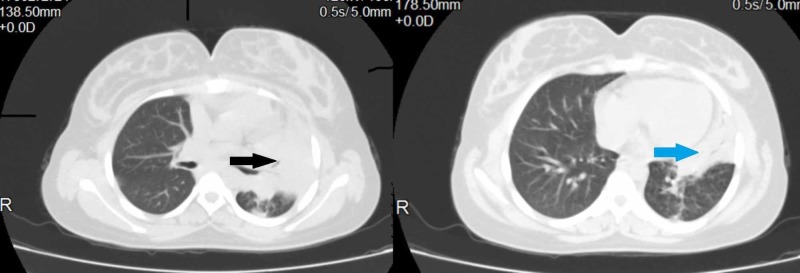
Computed tomography of the chest showing left upper lobe (black arrow) and lingular lobe (blue arrow) consolidation.
